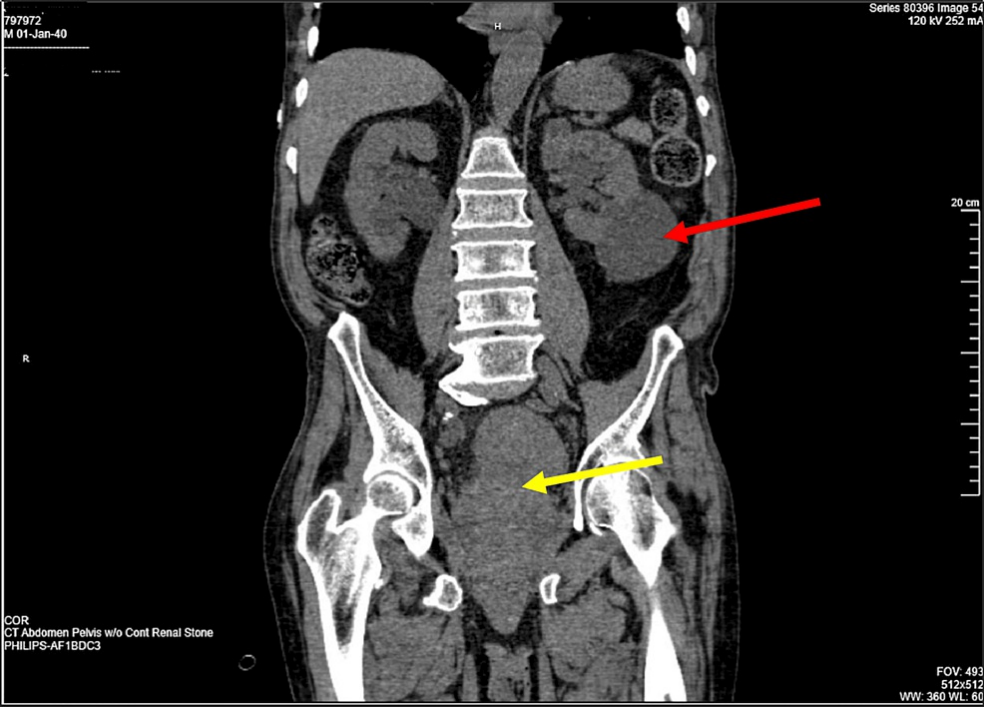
CT scan of abdomen and pelvis (coronal view) Moderate bilateral hydroureteronephrosis. Incidental finding of the left renal cyst within the lower pole (red arrow). Marked heterogeneity within the urinary bladder likely represents hemorrhage (yellow arrow)
